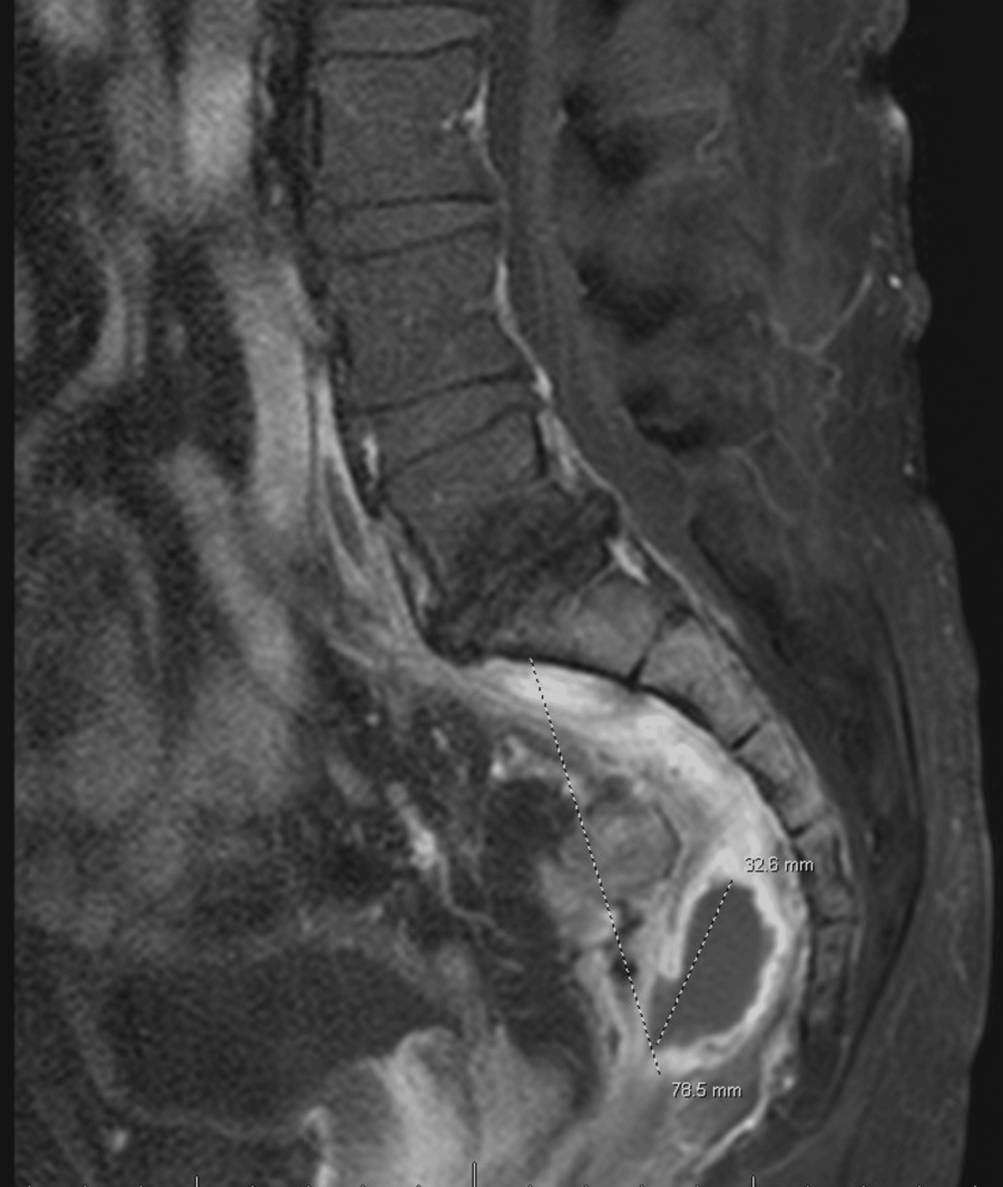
MRI sacrum showing edema and enhancement throughout the presacral region, compatible with a presacral abscess
